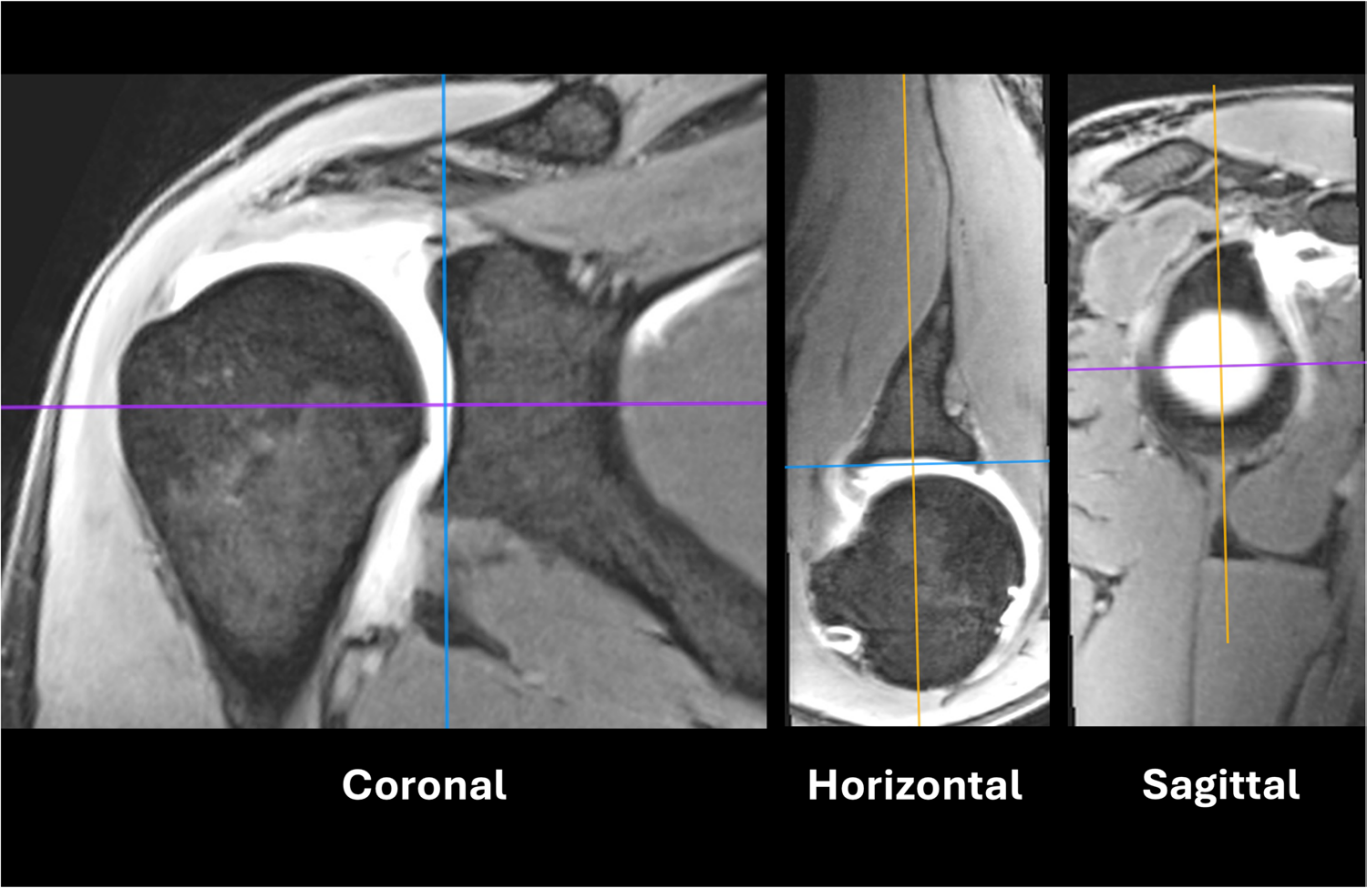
Multiplannar reconstruction based on VIBE sequence of MRI
